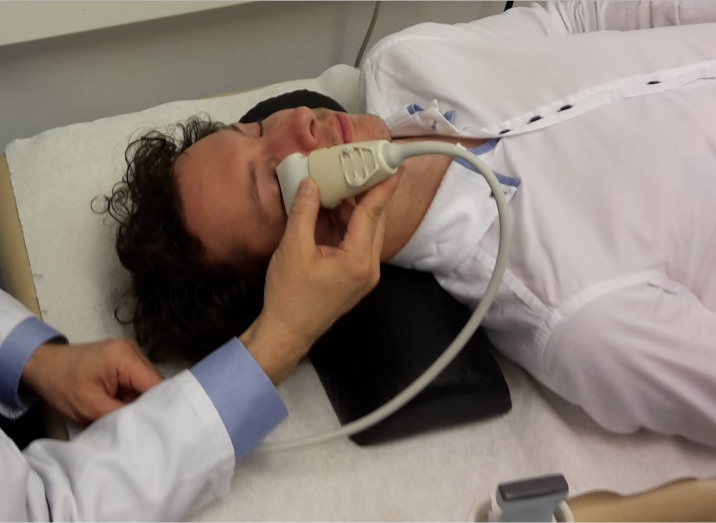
Assessment of pupillary function by B-mode ultrasound. Position of the proband and ultrasound probe.
